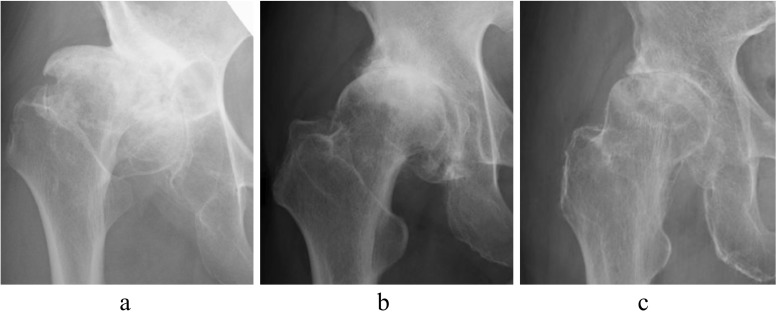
Radiographs of OA according to the Bombelli classification. Typical radiographs of hypertrophic (a), normotrophic (b), and atrophic (c) OA
